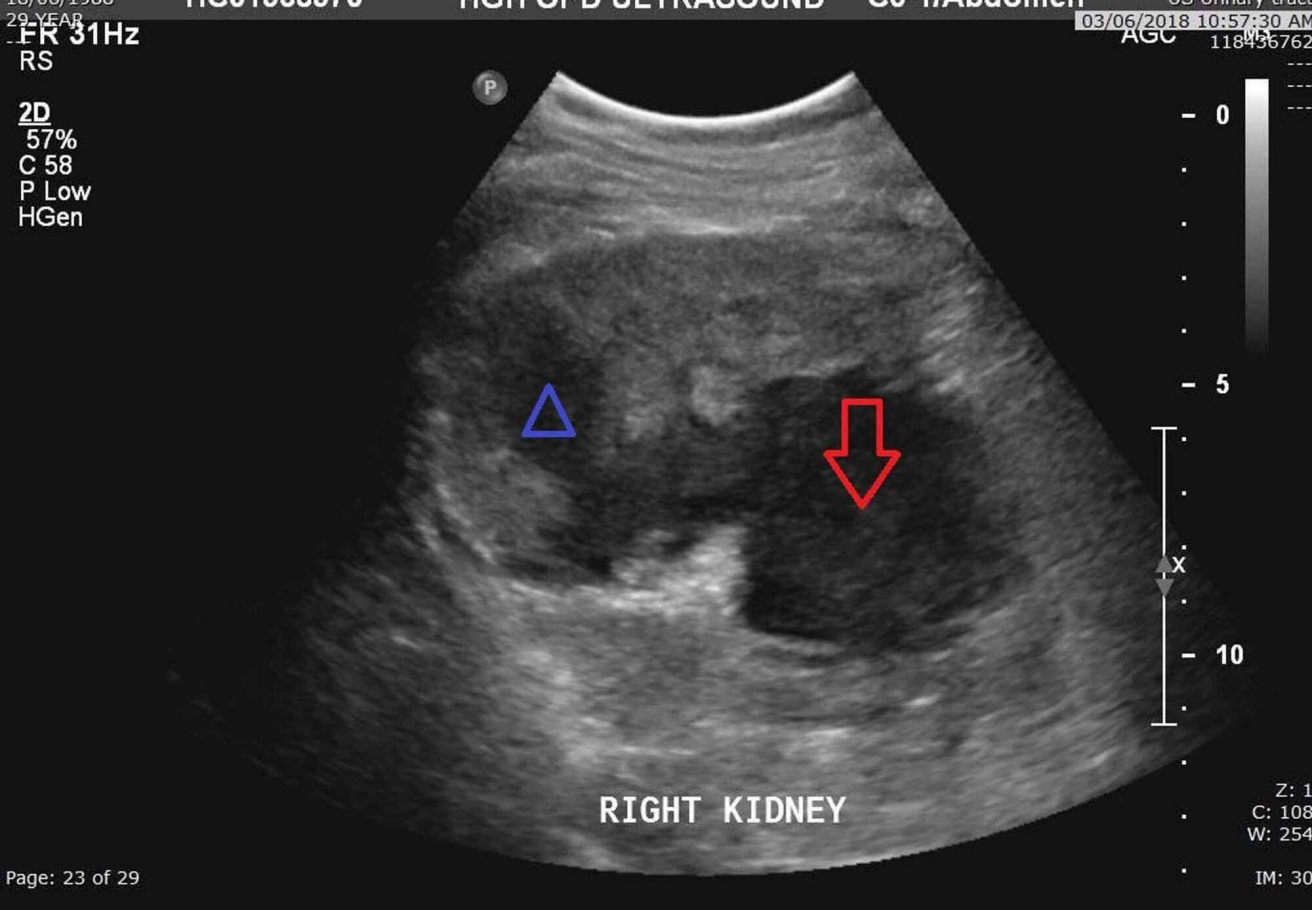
Ultrasound: showing perinephric hematoma (arrowhead) communicating with hemorrhagic cyst (arrow).
